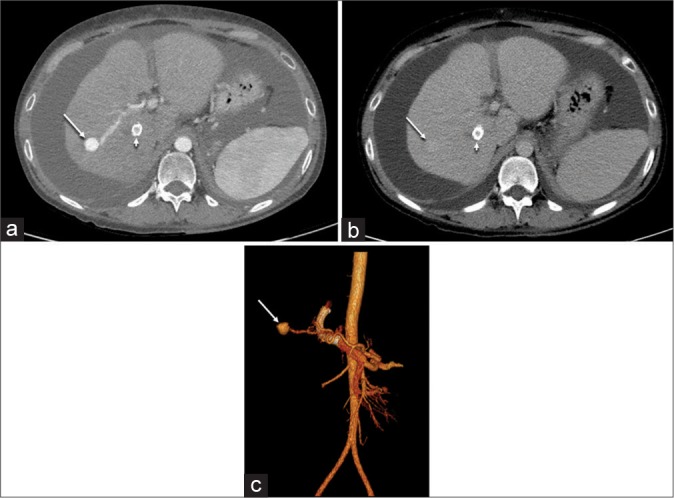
(a) Axial computed tomography angiography in the arterial phase demonstrate a round 1.5-cm lesion in the right hepatic lobe (arrow) with contrast enhancement that matches the arterial blood pool. The newly placed transjugular intrahepatic portosystemic shunt is also visible (arrowhead). (b) Axial contrast-enhanced computed tomography image in the delayed phase demonstrates a faintly enhancing lesion in the right hepatic lobe (arrow) with enhancement that matches the blood pool. The newly placed transjugular intrahepatic portosystemic shunt is also visible (arrowhead). (c) Volume-rendered image from computed tomography angiography demonstrates the hepatic artery pseudoaneurysm (arrow).
